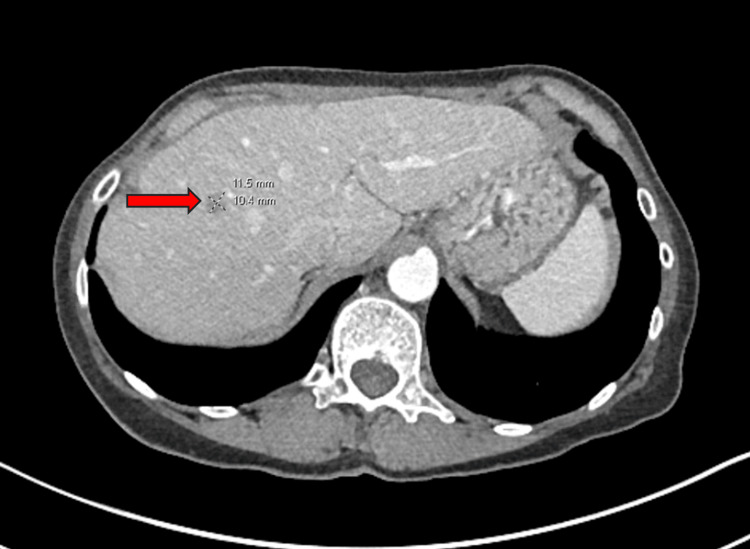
Axial CT showing low-attenuation nodule in the dome of the liver from May 2022. CT: computed tomography
